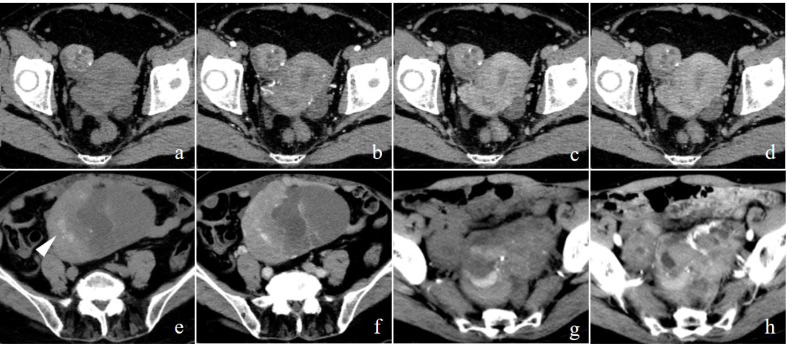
Three cases of BTs with CT and MRI scans. (a–d). A 55-year-old woman diagnosed with a benign BT, characterized by a well-defined, mainly solid mass with irregular calcification and mild progressive enhancement on CECT scan. (e, f). Borderline Brenner tumor in a 75-year-old woman. Amorphous clustered calcifications (arrow) and relatively prominent papillary projections are visible. (g, h). A 71-year-old woman with a malignant BT, identified by an irregular mass containing hemorrhagic components, coarse calcifications, and pronounced enhancement.
